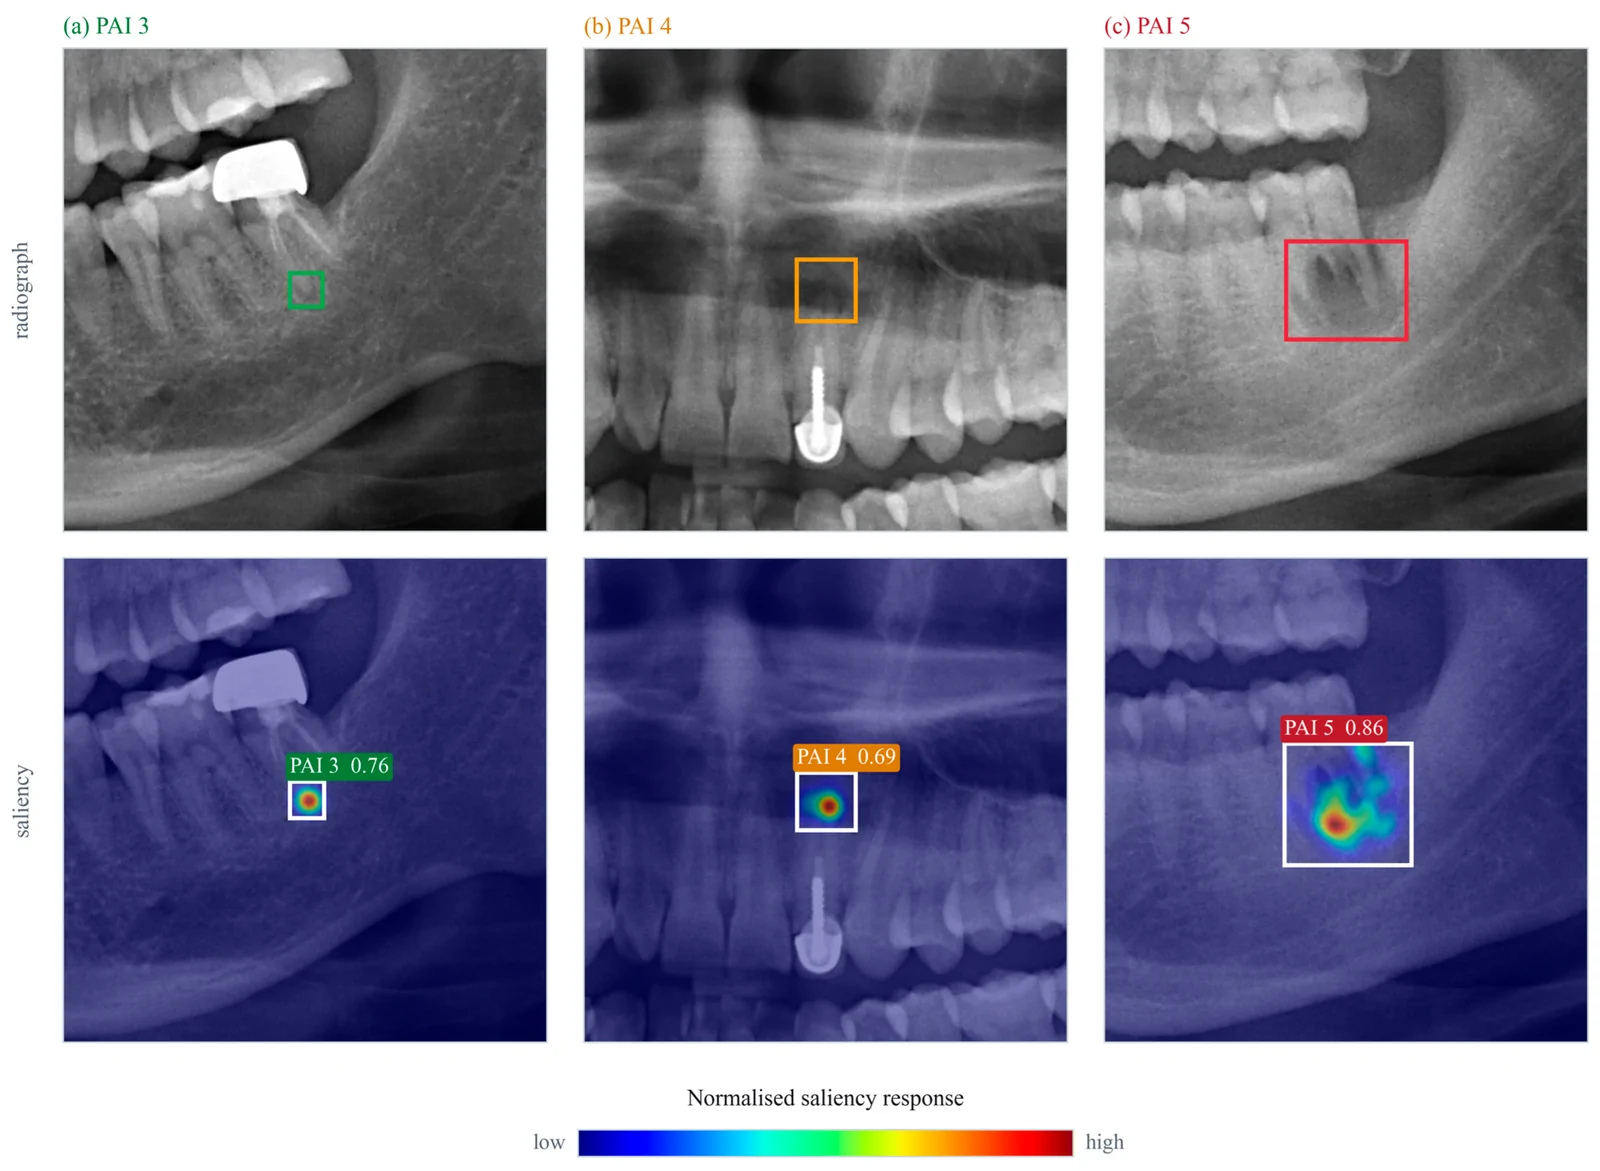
Saliency of the stride-4 P2 level that feeds the detection head, for one correctly detected and correctly graded lesion of each grade: (a) PAI 3, (b) PAI 4 and (c) PAI 5. The upper row is the radiograph with the reference annotation outlined in the colour of its grade, and the lower row the same field with the saliency map overlaid and the model prediction drawn in white with its confidence. The map is the element-wise product of the P2 activation and its gradient with respect to the summed class score of every anchor inside the reference lesion, read before non-maximum suppression, normalised to its maximum and smoothed for display only.
